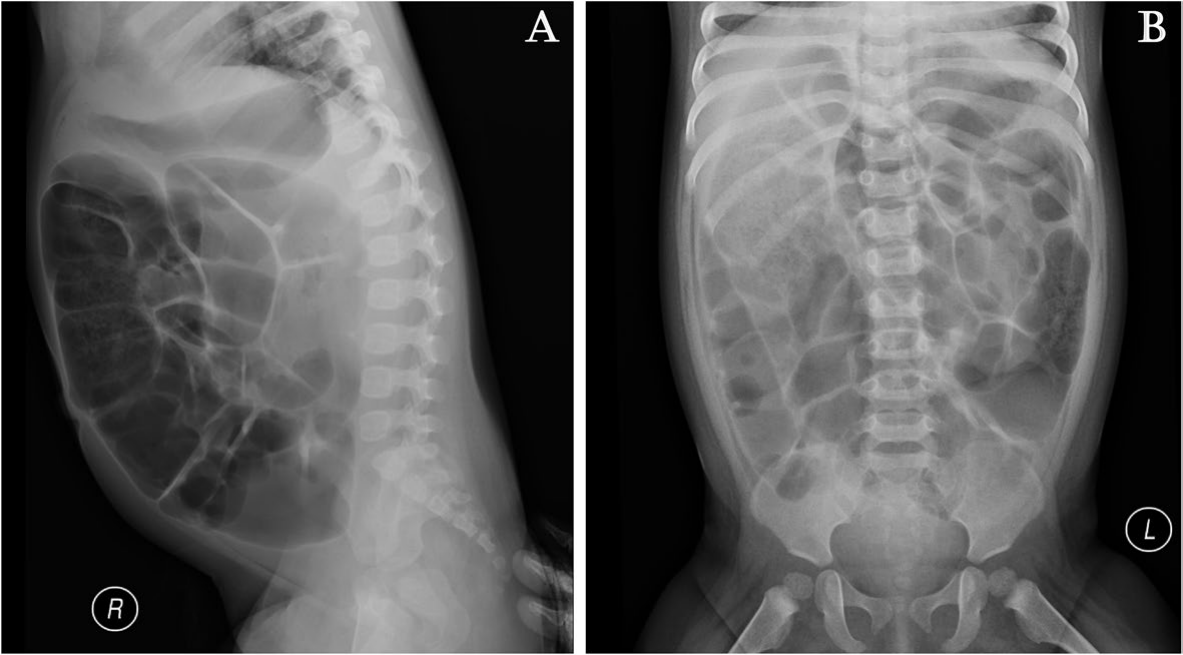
At 1 year of age, abdominal X-ray revealed mechanical ileus. A Right lateral abdominal radiograph; B  Supine abdominal radiograph
